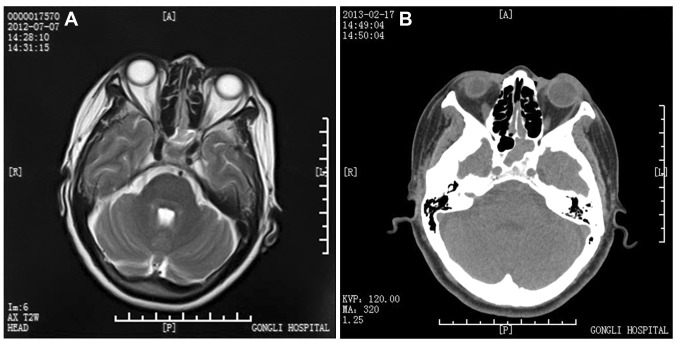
Axial magnetic resonance imaging/computed tomography (MRI/CT) scan. (A) Axial T2 weighted MRI scan of the head revealing a soft tissue mass situated exclusively in the bilateral olfactory cleft area, not invading other sinuses, orbit, or the bone structure of the skull base. (B) A 64-slice spiral axial CT plain scan of the paranasal sinus in the same area described in A.
